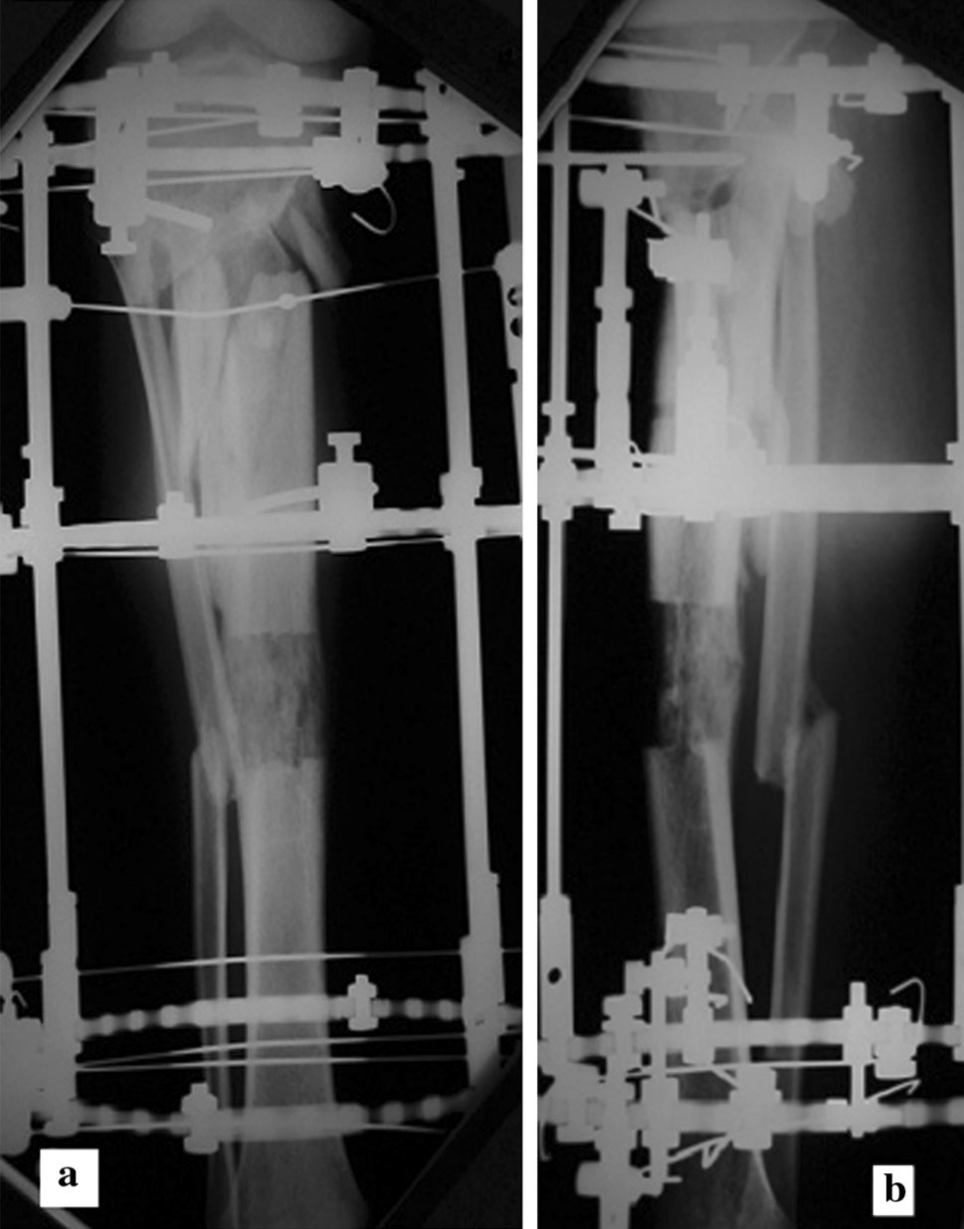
a, b Antero-posterior and lateral radiographs showing the distracted segment bridging the comminution gap, with good consolidation
